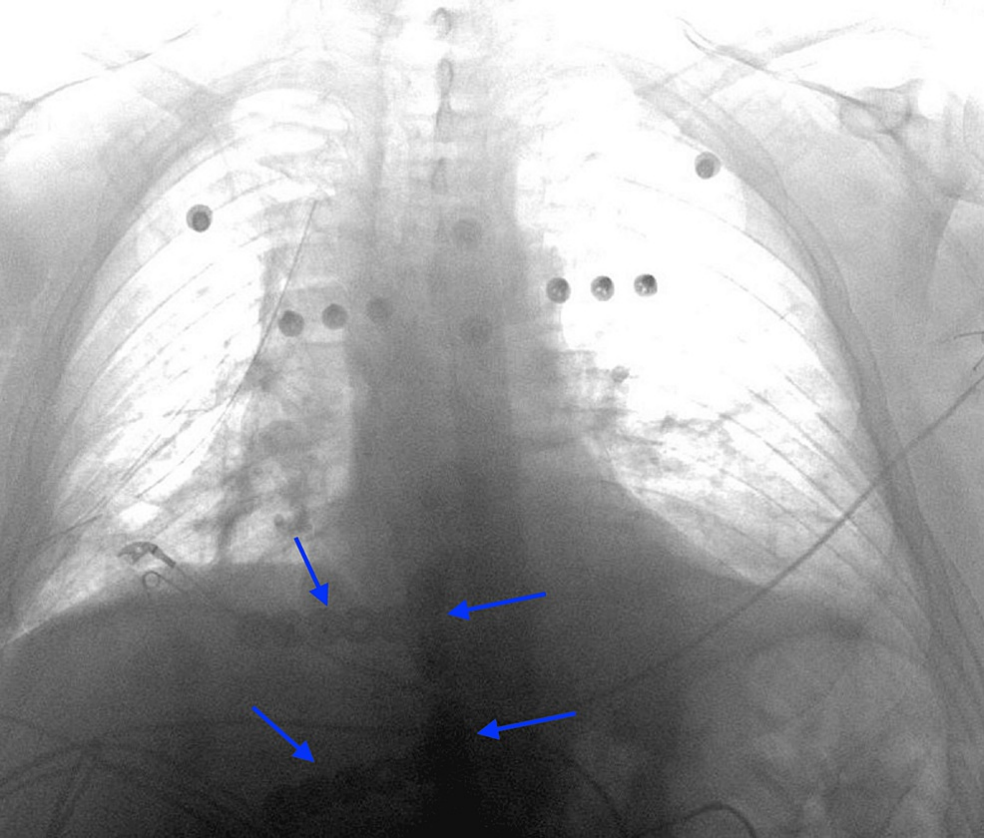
Postoperative chest radiograph showing titanium plates (blue arrows).
